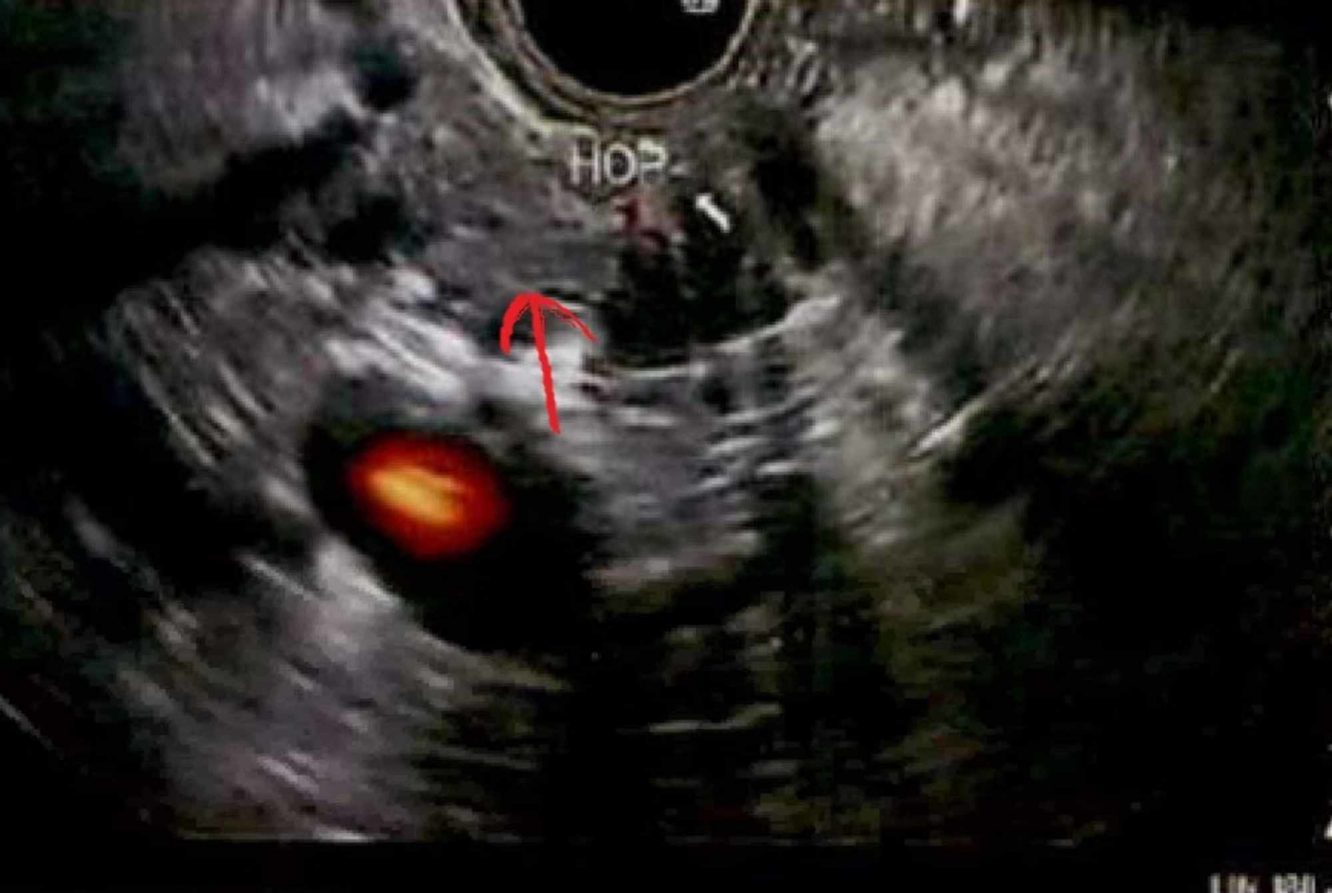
Endoscopic ultrasound showing hypoechogenic mass in the pancreatic body
